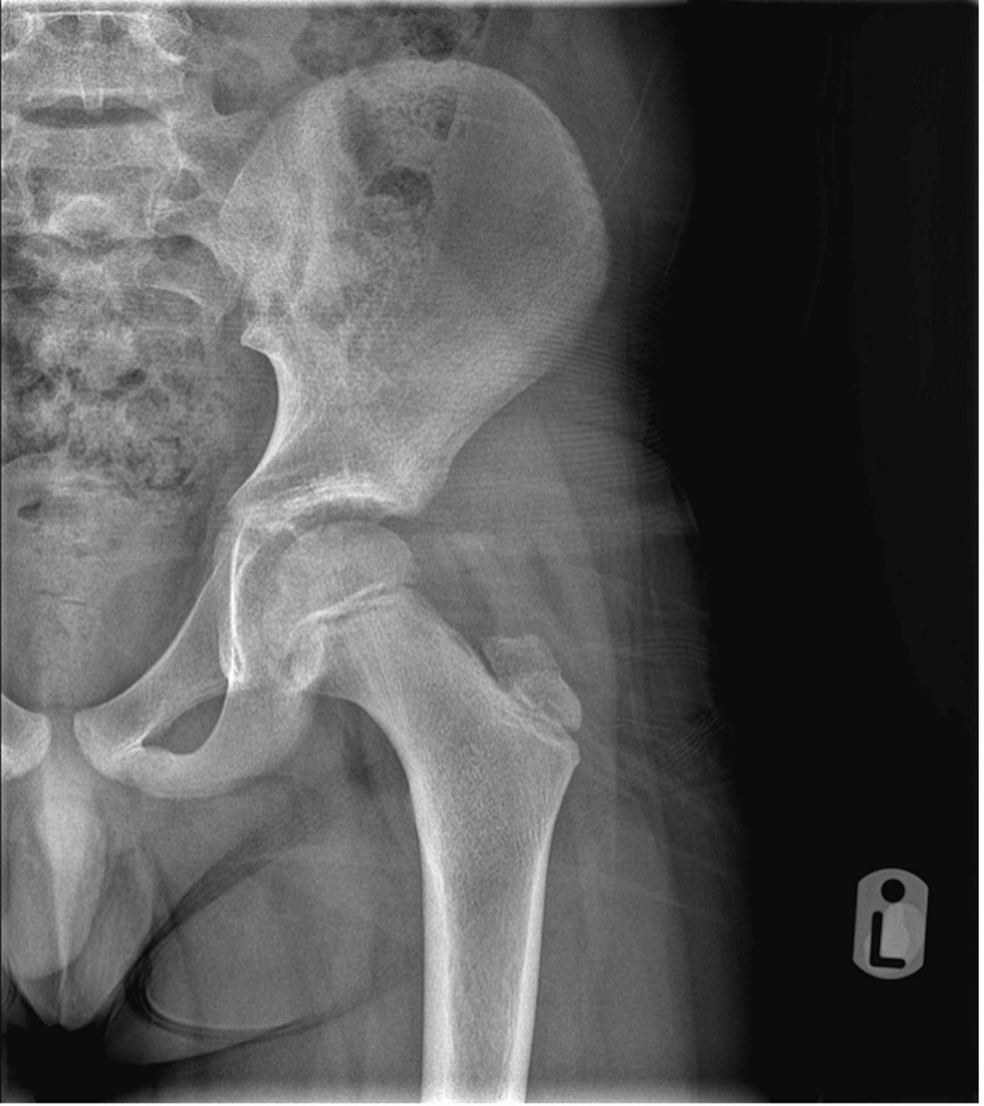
Follow-up X-Ray at 12 months without any radiological evidence of AVN.
